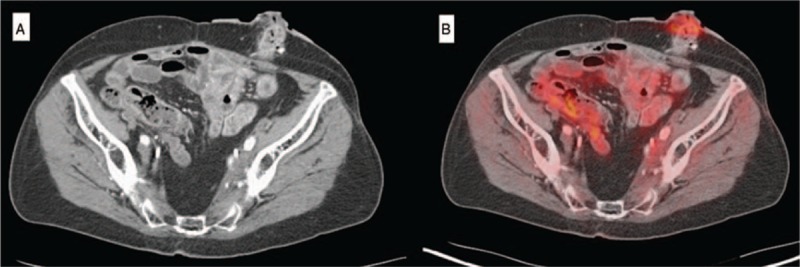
Pos-trituximab imaging revealing an unchanged soft tissue mass on CT (A) but substantially reduced FDG avidity on PET (Comparison Figure 9 A and B).
